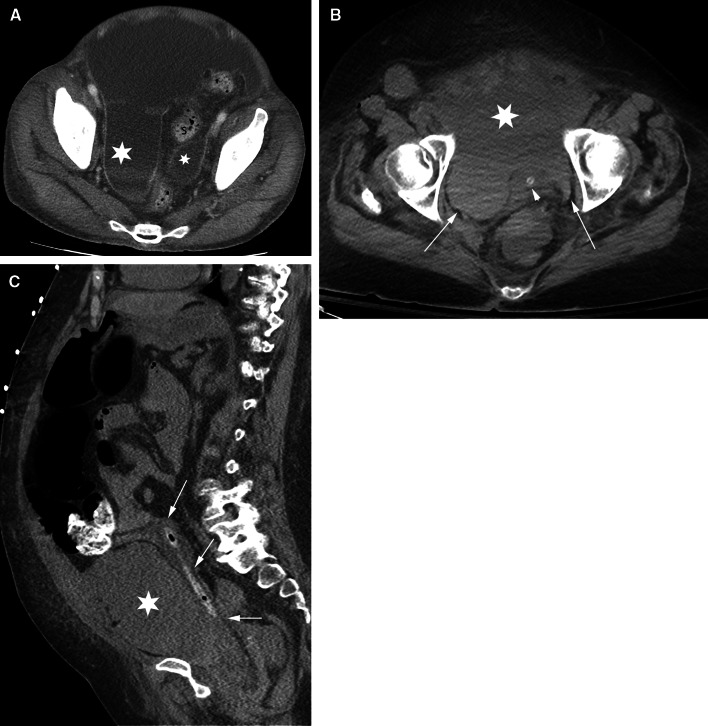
Pelvic intraperitoneal fluid vs extraperitoneal fluid. Pelvic fluid is shown in 2 different patients. The patient in A has intraperitoneal fluid. The patient in B and C has extraperitoneal fluid. A axial CT image shows intraperitoneal fluid in the right and left paravesical recesses (*asterisks*) lateral to the sigmoid colon (S) and superior to the bladder (not shown). B Axial CT image of a different patient shows extraperitoneal fluid in the prevesical space (*asterisk*) anterior to the decompressed bladder (*arrowhead*) containing a catheter. The bladder is displaced posteriorly. Fluid extends within the prevesical space (*arrows*) lateral to the bladder giving a “molar tooth” appearance. C sagittal CT image in the same patient as in B shows extraperitoneal fluid in the prevesical space (*asterisk*) anterior to the posteriorly displaced bladder (*arrows*) containing a catheter.
